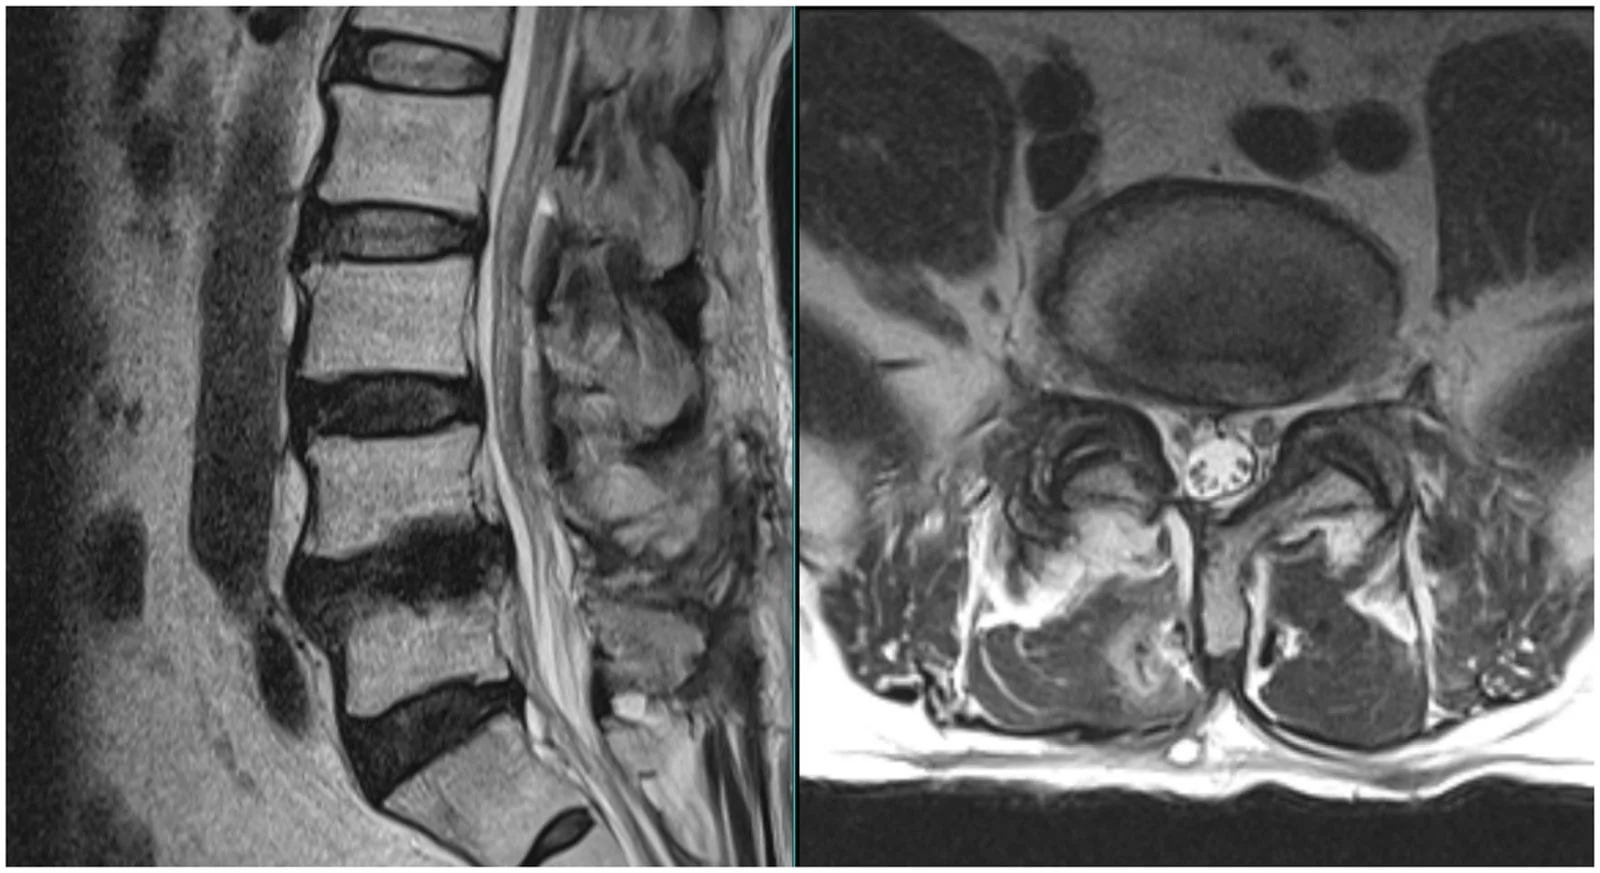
Follow-up lumbar spinal MRI showing resolution of epidural hematoma. MRI = magnetic resonance imaging.
